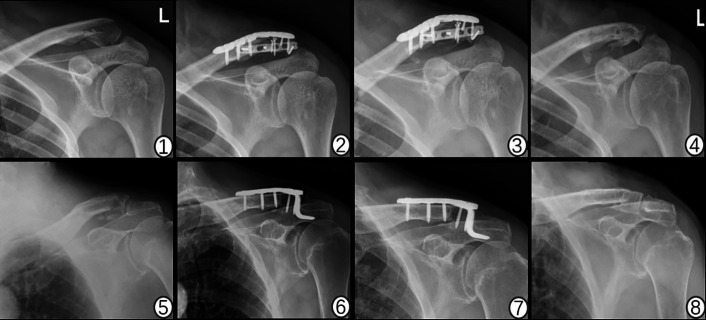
Radiographic evaluation of distal clavicle fractures treated with clavicular hook plate versus vertical double-plate. ①⑤Preoperative radiographs of Neer type V distal clavicle fractures. ①A 53-year-old male patient; ⑤A 76-year-old female patient. ②⑥Radiographs at 1 month after surgery show treatment with clavicular hook plate versus vertical double-plate.③⑦Radiographs at 3 months after surgery show good fracture healing and alignment.④⑧Postoperative radiographs at 1 year after hardware removal.
